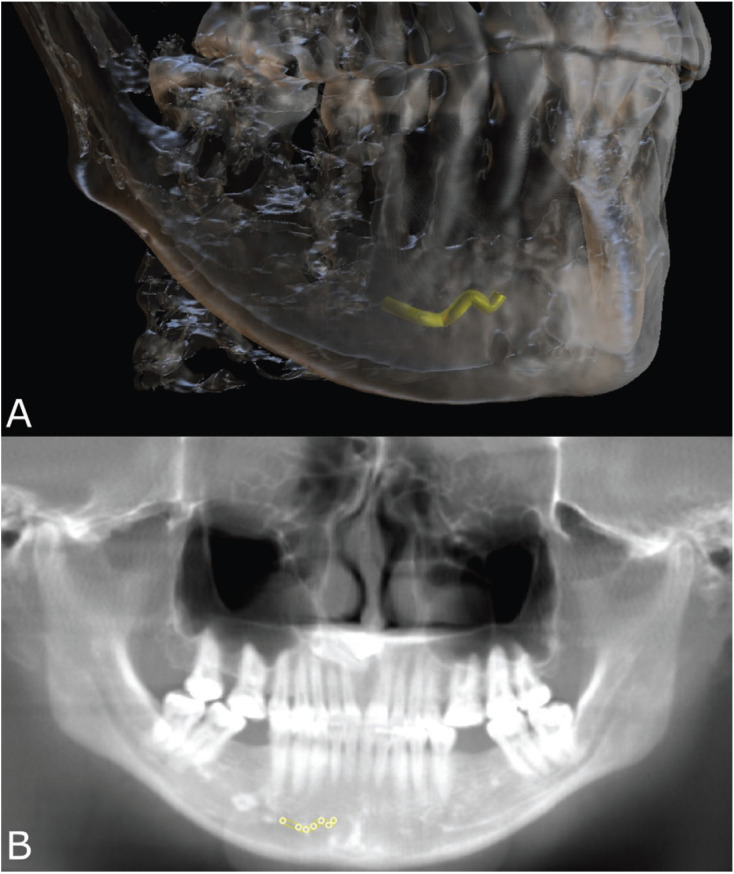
3D A) and panoramic B) reconstructions of a cone beam computed tomography volume showing the mandibular incisive canal (MIC) bone path (yellow) in the interforaminal region of the anterior portion of the mandible.
